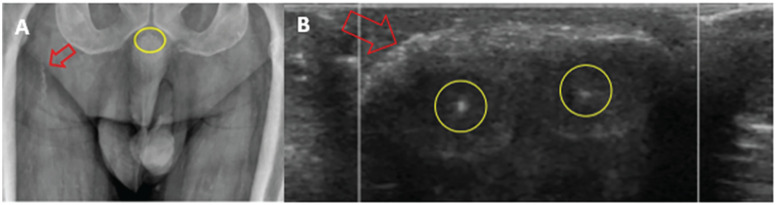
Imaging findings supportive of the diagnosis of calciphylaxis. Plain radiograph of pelvis (subfigure 2A) revealing calcifications in the vascular structures at the base of the penis (yellow circle) and calcified vessel in upper right anterior thigh (red arrow). US of glans penis (subfigure 2B) revealing calcification of both penile arteries (yellow circles) and dense curvilinear calcification of the glans penis (red arrow).
